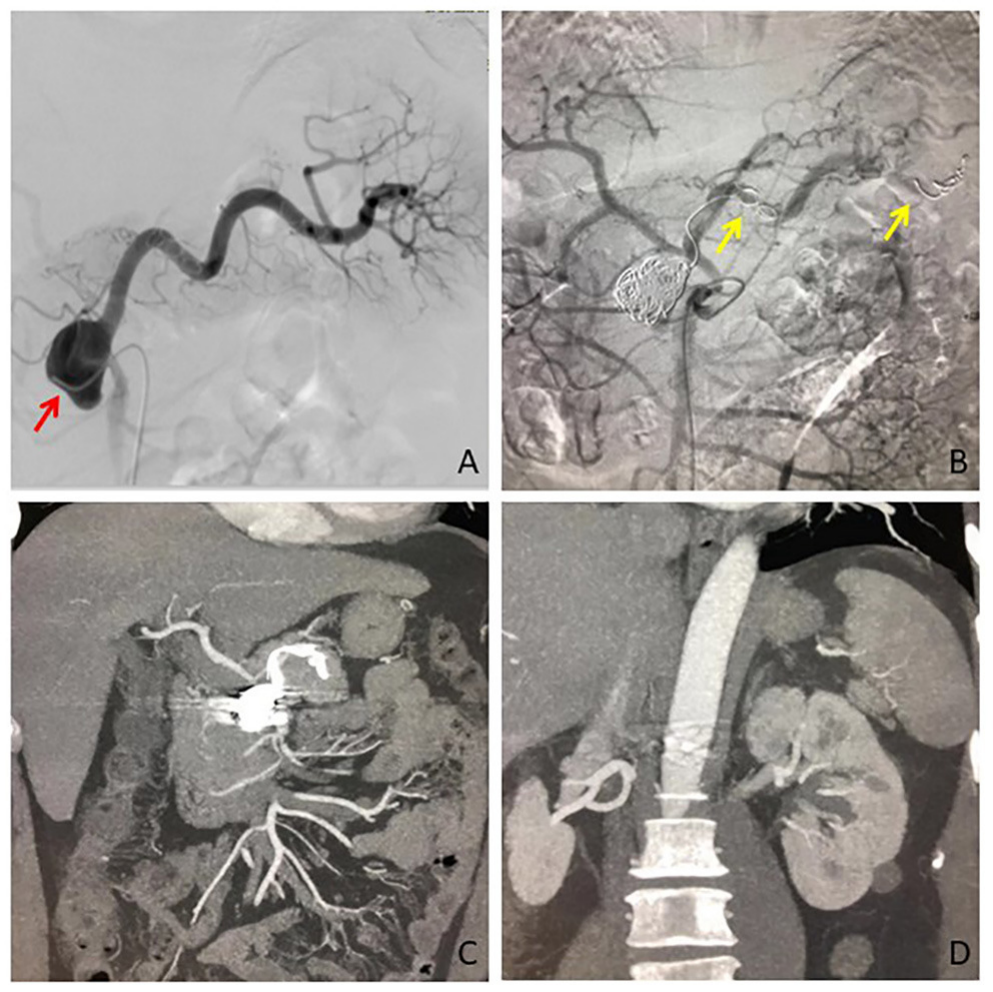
The SAA was diagnosed in a patient with the symptom of abdominal discomfort. (A) Digital subtraction angiography confirmed the location of SAA at the initiation of the splenic artery, which arose from the superior mesenteric artery (red arrow). (B) The distal side of the SAA parent artery, aneurysm sac, and the proximal side was embolized by coils (yellow arrow) in sequence. (C) CT angiography at 3 months showed completely aneurysmal thrombosis, and (D) No splenic infarction occurred. SAA, splenic artery aneurysm.
